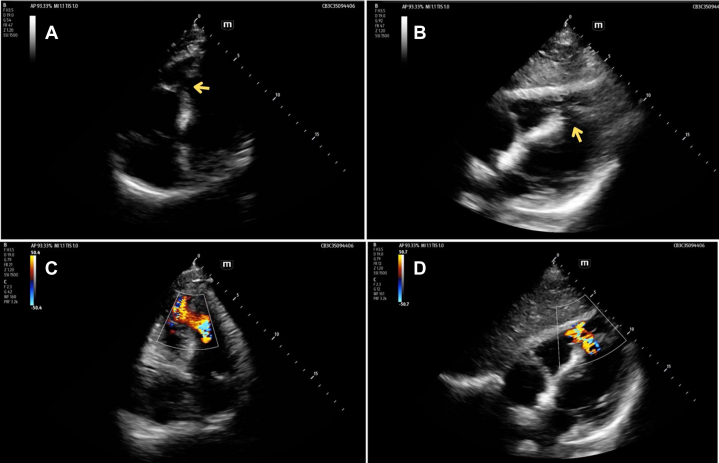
Point-of-care focused cardiac ultrasound was performed on a patient with elevated, high-sensitivity troponin after blunt chest wall trauma. The apical 4 (A) and subxiphoid (B) views show an apical ventricular septal defect (yellow arrow), apical hypokinesis, preserved ejection fraction, and equality of ventricles. Color Doppler shows turbulent flow through the defect (C and D).
